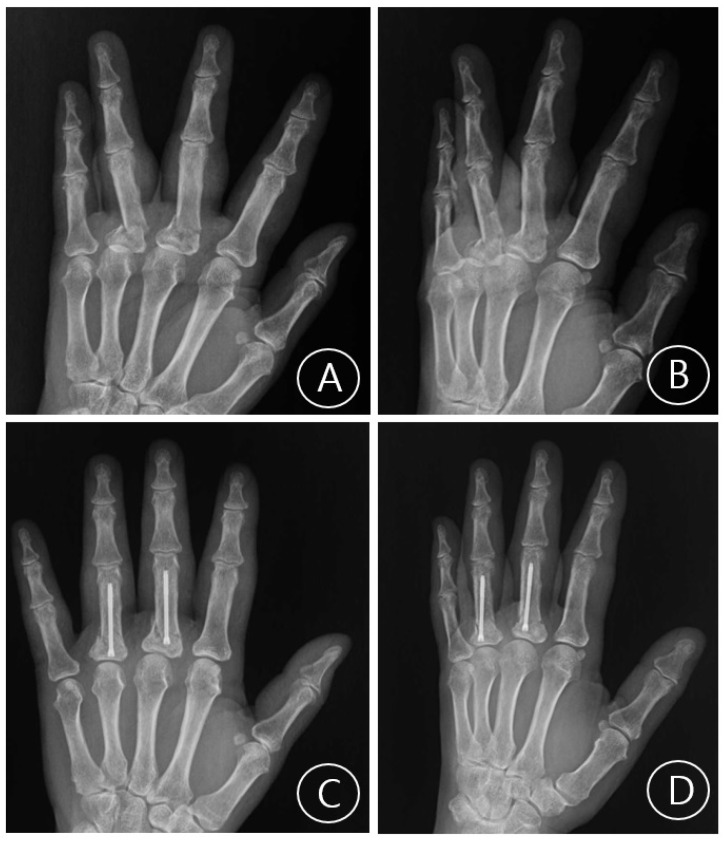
A case of a left third and fourth digit transverse proximal phalangeal fractures. (A) Preoperative plain radiograph Posteroanterior view. (B) Preoperative plain radiograph oblique view. (C) Postoperative plain radiograph Posteroanterior view taken at last follow-up visit. (D) Postoperative plain radiograph oblique view taken at last follow-up visit.
